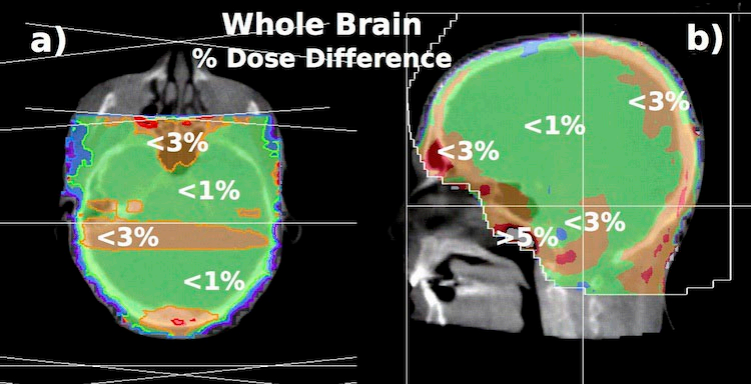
Dose difference for a whole‐brain plan: (a) axial view and (b) sagittal view of the relative dose difference of a whole‐brain treatment with opposed lateral beams. The displayed dose difference is relative to the CT planned dose distribution. The plan used MLCs to shape the PTV.
